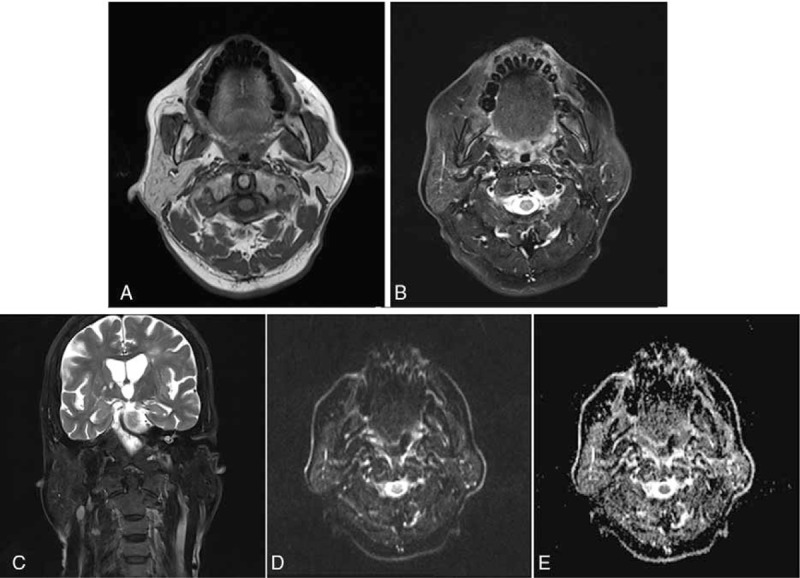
Parotid MRI and DWI. Representative MRI images showing the T1WI axial (A), T2WI axial (B), and T2WI coronal views (C). DWI (D) and ADC (E) images. ADC = apparent diffusion coefficient, DWI = diffusion-weighted magnetic resonance imaging, MRI = magnetic resonance imaging.
